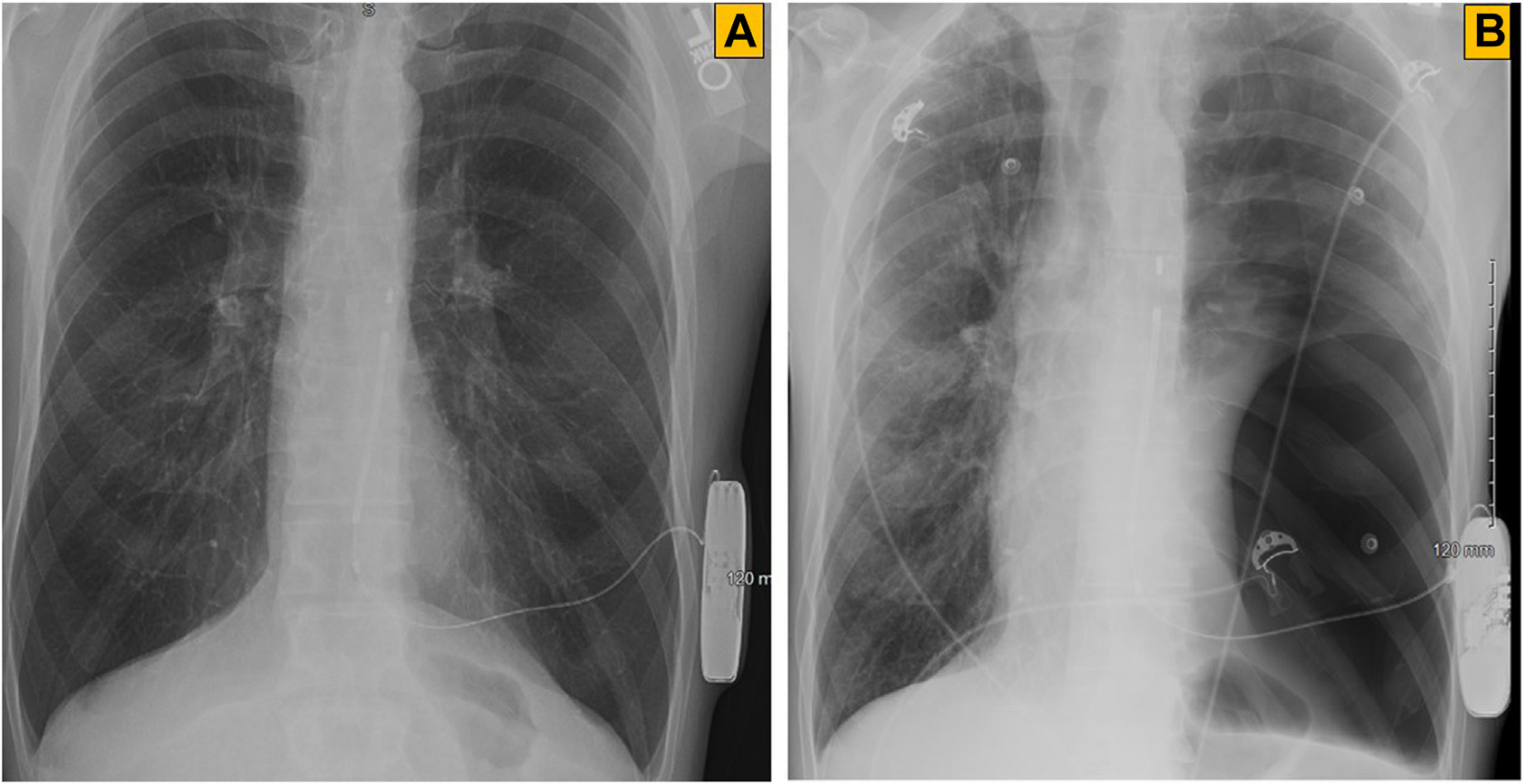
Chest radiographs, posteroanterior views. A: Obtained at subcutaneous implantable cardioverter-defibrillator implantation, showing hyperinflated lungs with radiolucent areas bilaterally, more pronounced on left side. B: At device malfunction, showing progressive disease, traction bronchiectasis, and expansion of emphysematous bullae.
